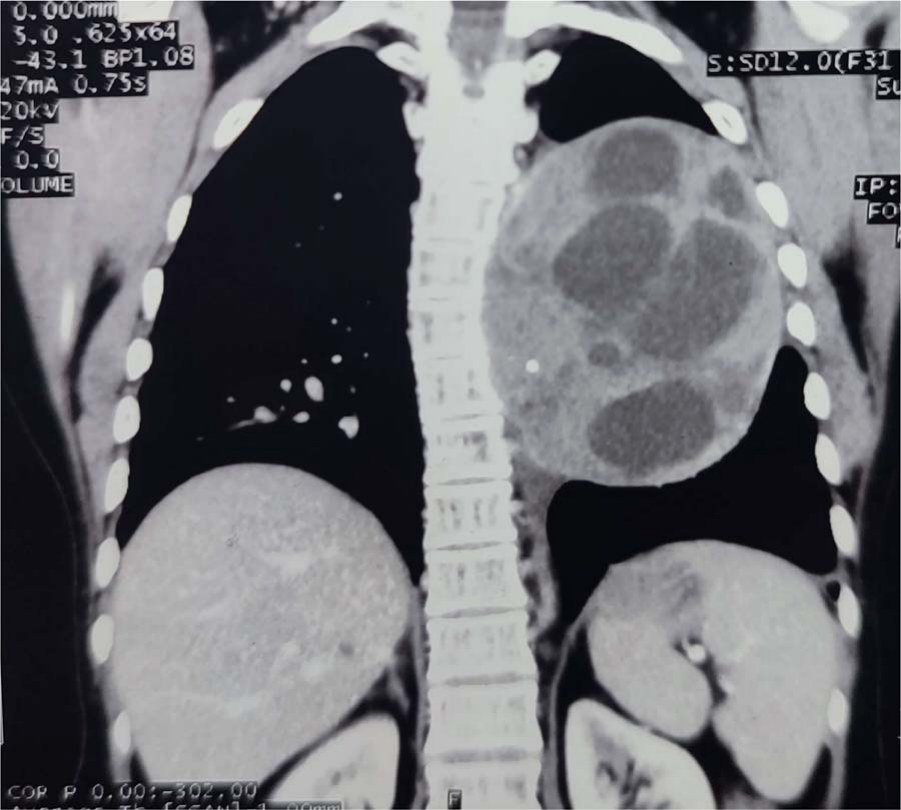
Contrast-enhanced CT of the chest demonstrates a large, well-defined, multiloculated cystic lesion in the left lower lobe of the lung, measuring approximately 11.2 × 9.8 × 11.6 cm. The lesion contains multiple internal septations and daughter cysts with areas of calcification. It causes mass effect, displacing the left main pulmonary artery, left main bronchus, descending aorta, and esophagus.
